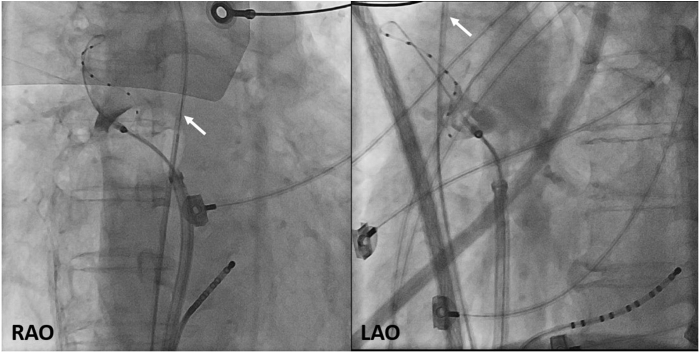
RAO and LAO radiographs showed grade 4 occlusion of the right superior pulmonary vein during cryoballoon ablation. The pacing catheter (*white arrow*) is positioned deep in the superior vena cava at a site where consistent phrenic nerve stimulation is achieved. LAO = left anterior oblique; RAO = right anterior oblique.
